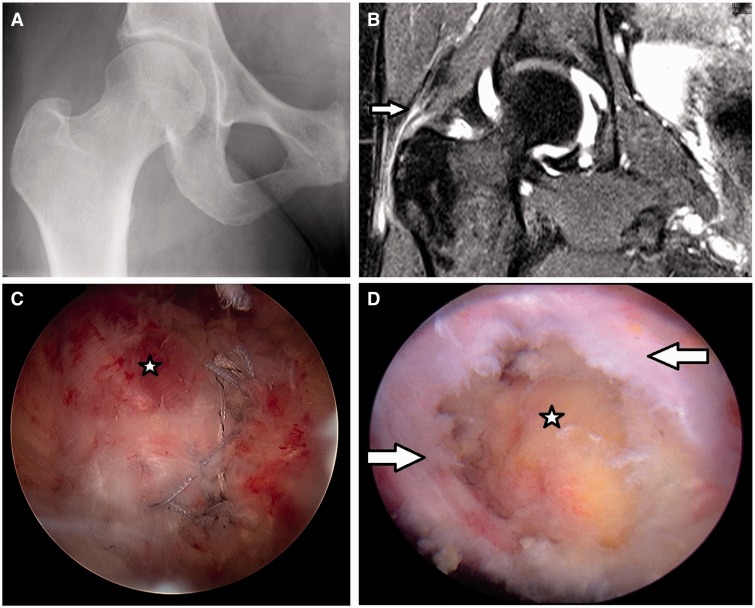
(A) X-ray of a 55–year-old patient with partial GM tear without significant findings; (B) MRA in T2 fat supression protocol of the same patient, arrow pointing to GM tendon partial thickness tears. In that case the radiologist interpeted as resolving tendinosis, while the surgeon as partial GM tear; (D) Arthroscopic image of a debrided partial GM tear, the arrows pointing at the tear, the star located at the bald trochanter; (C) The repaired tendon.
